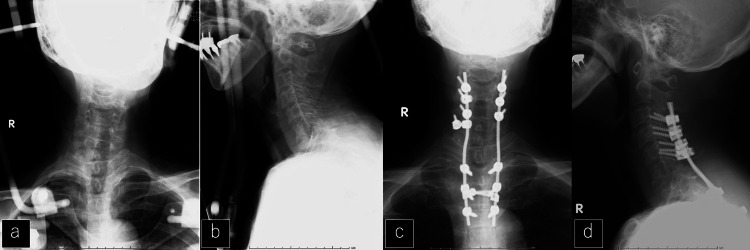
X-ray after halo vest immobilization and posterior fixation a, b: X-ray after temporal halo vest immobilization. c, d: X-ray after posterior fixation.
